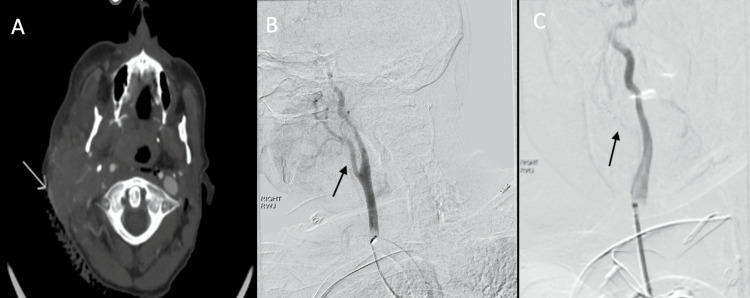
CTA of head/neck with contrast pooling at a superficial aspect of tumor posteriorly (A); initial common carotid digital subtraction image demonstrates that the right external carotid artery supplies a hypervascular tumor (B); after coil embolization of the external carotid, no tumoral enhancement is evident (C, enlargement of image B)
